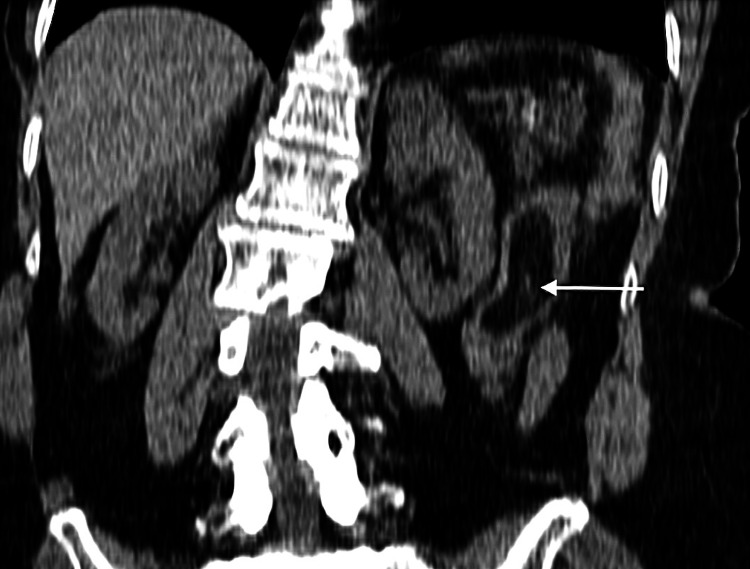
Reformatted coronal noncontrast CT through the upper abdomen showing intraluminal fat density within the jejunum (horizontal arrow)
